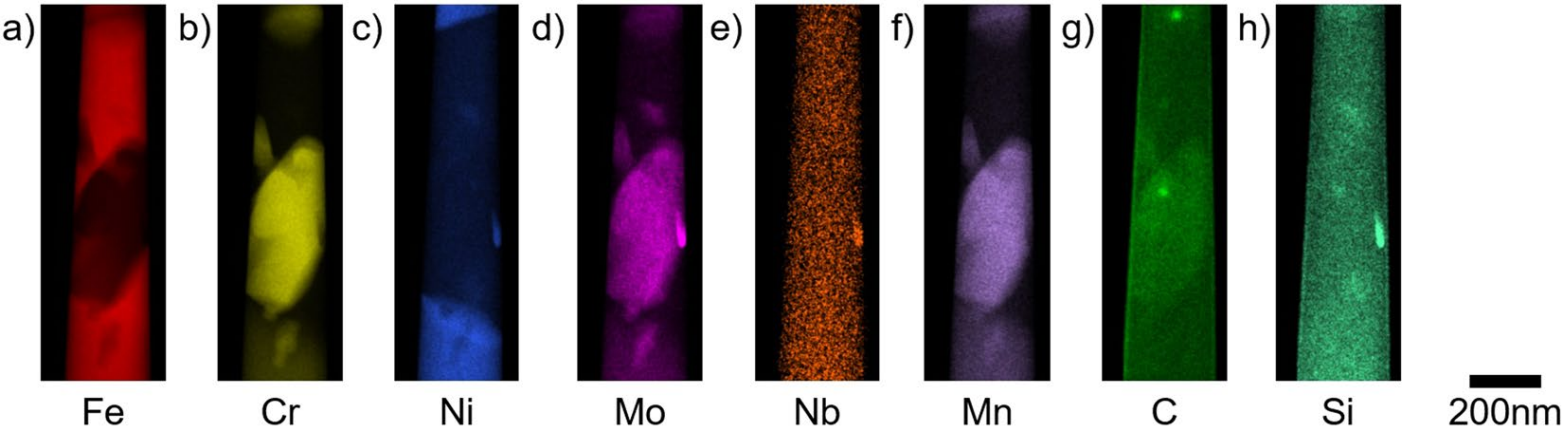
STEM-EDX elemental maps of the STEM needle displaying the intensity of X-ray counts associated with the (a) Fe Kα, (b) Cr Kα, (c) Ni Kα, (d) Mo Lα, (e) Nb Kα, (f) Mn Kα, (g) C Kα and (h) Si Kα peaks. The austenite phase at top and bottom is associated with Fe, Ni and to a lesser extent Mo and Mn, the ferrite phase is predominantly Fe with a small amount of Ni and Cr, the carbides contain Cr, Fe, Mo, C, Ni and Mn, the G-phase precipitate contains Nb, Ni, Mo and Si. The surface of the needle has high carbon and silicon intensities that are associated with organic contamination, primarily due to long exposure under the electron beam.
